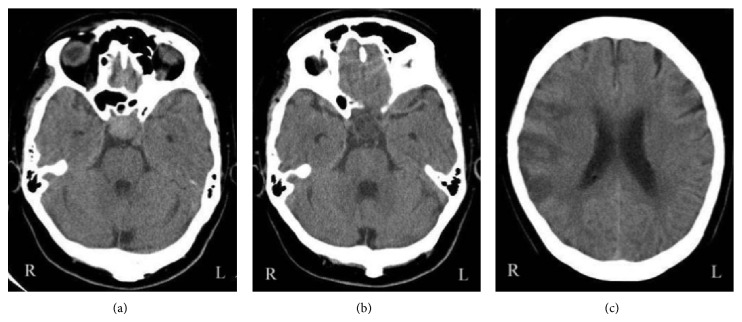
(a) Axial view of the CT showing the round and slightly hyperdense area in the suprasellar region at the first emergency visit. (b, c) Axial view of the CT showing the round hypodense area in the suprasellar region and the low density area in the territory of the right middle cerebral artery at the second emergency visit.
